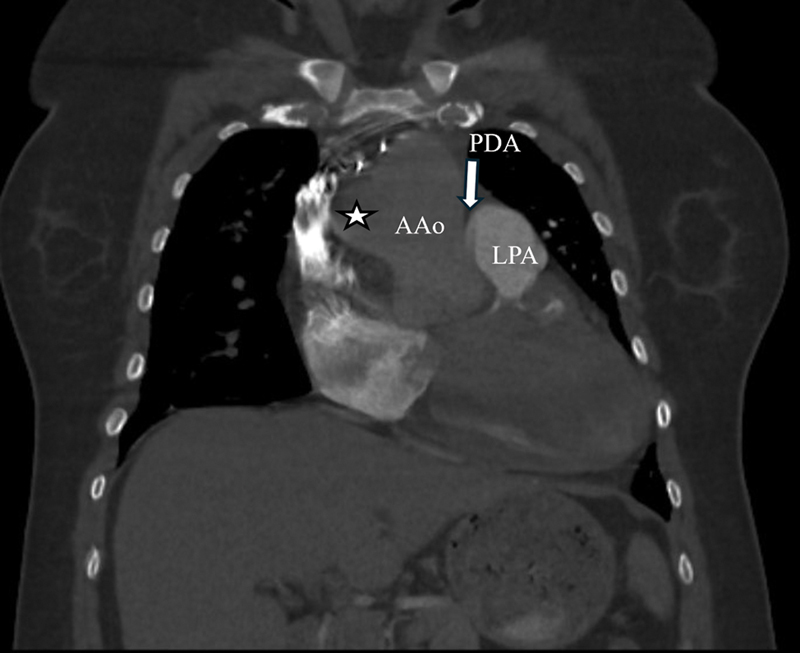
Early phase coronal computed tomography angiography (CTA) shows anomalous origin of the right pulmonary artery (RPA, star) from the ascending aorta (AAo). The left pulmonary artery (LPA) is better opacified than the aorta. Also noted is the patent ductus arteriosus (PDA, arrow).
